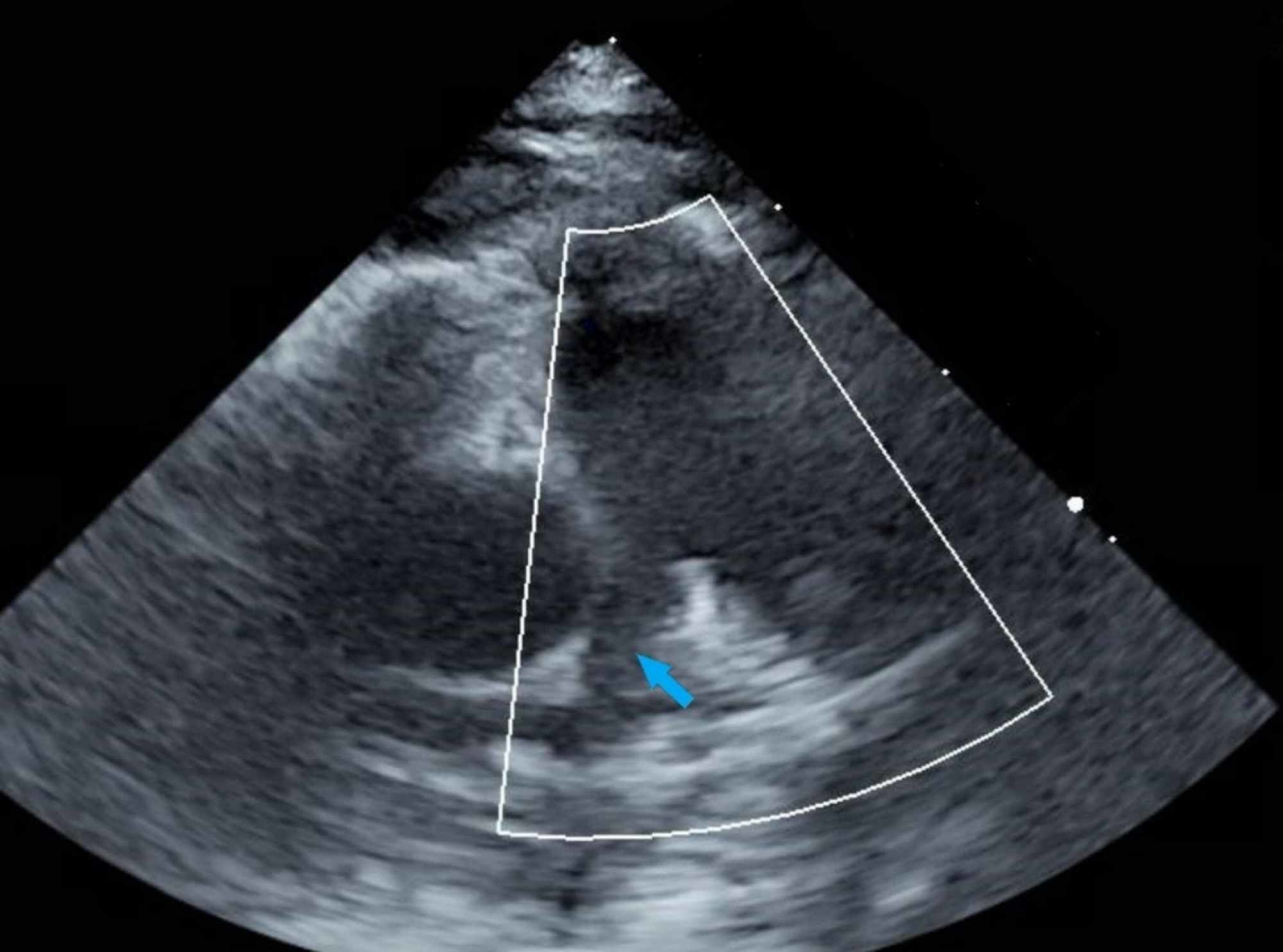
Echocardiogram parasternal short axis view showing peripheral pulmonary artery stenosis (arrow).
